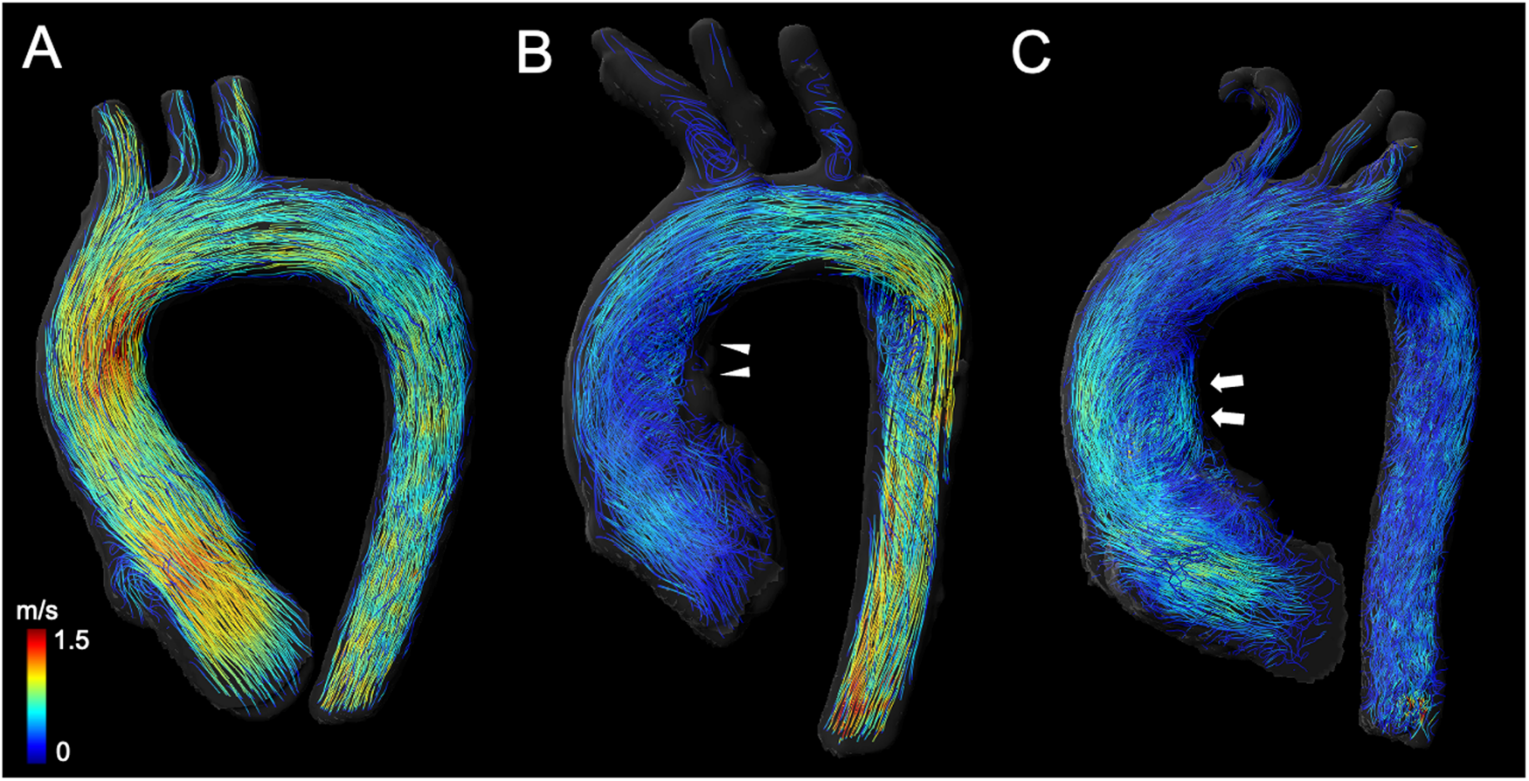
4D flow MRI-based streamline visualization of hemodynamics in the thoracic aorta of Marfan patients according to the three identified clusters. A Physiological blood flow in a 41-year-old female Marfan patient from cluster 1 with a Z-score of 0.3. B Moderate *helical* (grade 1) blood flow in a 20-year-old female Marfan patient from cluster 2 with a Z-score of 3.4. C Pronounced combined *helical* (grade 2) and *vortical* (grade 2) blood flow in a 60-year-old female Marfan patient from cluster 3 with a Z-score of 4.0
